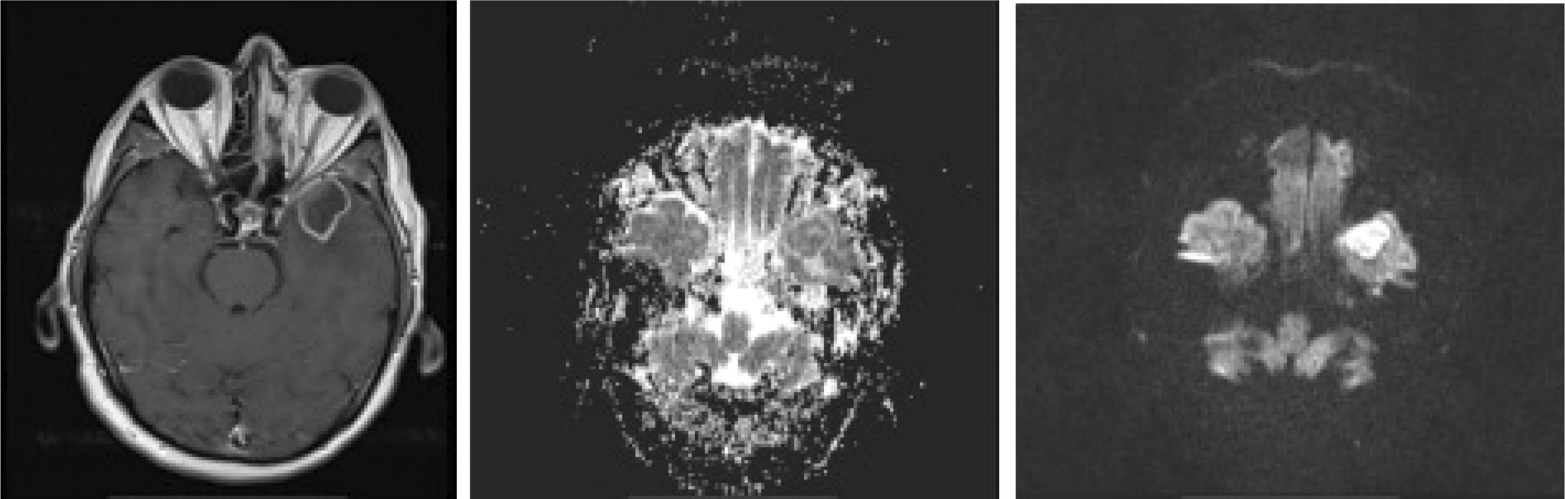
Rim enhancing abscess in left temporal lobe with restriction in DWI sequence.
